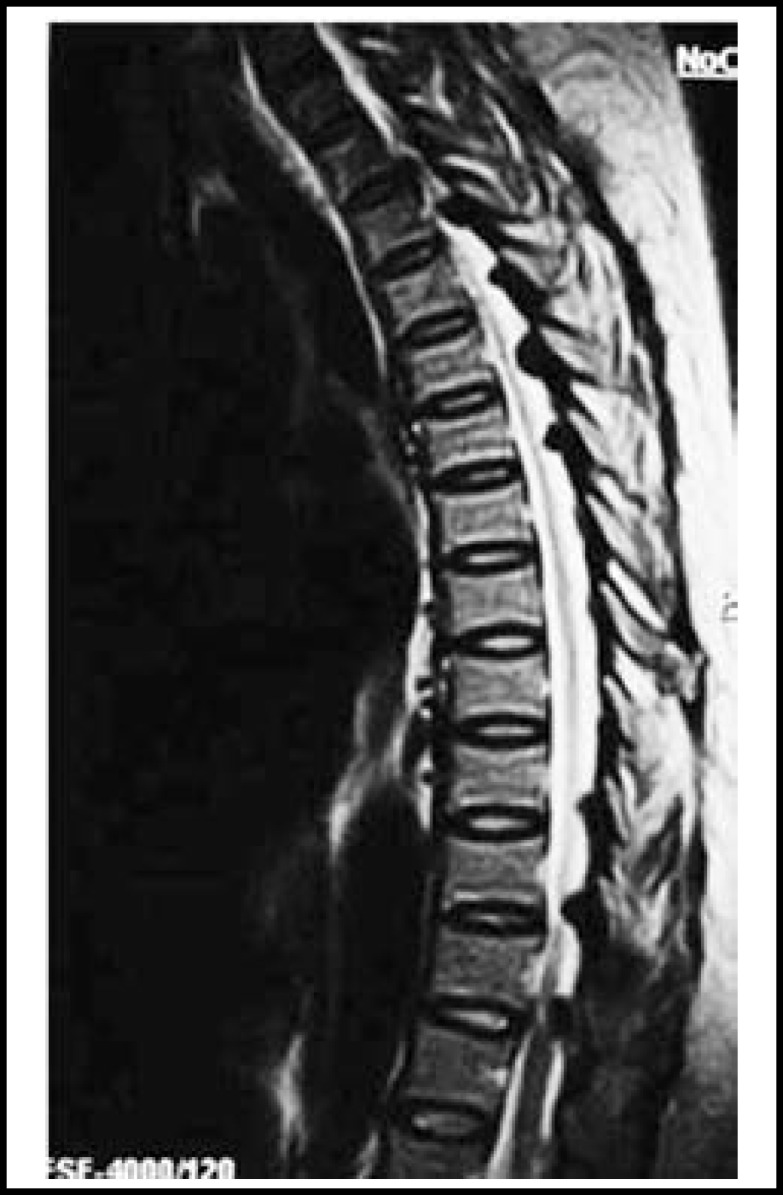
T2 weight MRI of thoracic spine showed thickening of anterior and posterior longitudinal ligaments between T3–T7 and T10–L1 (case 17).
